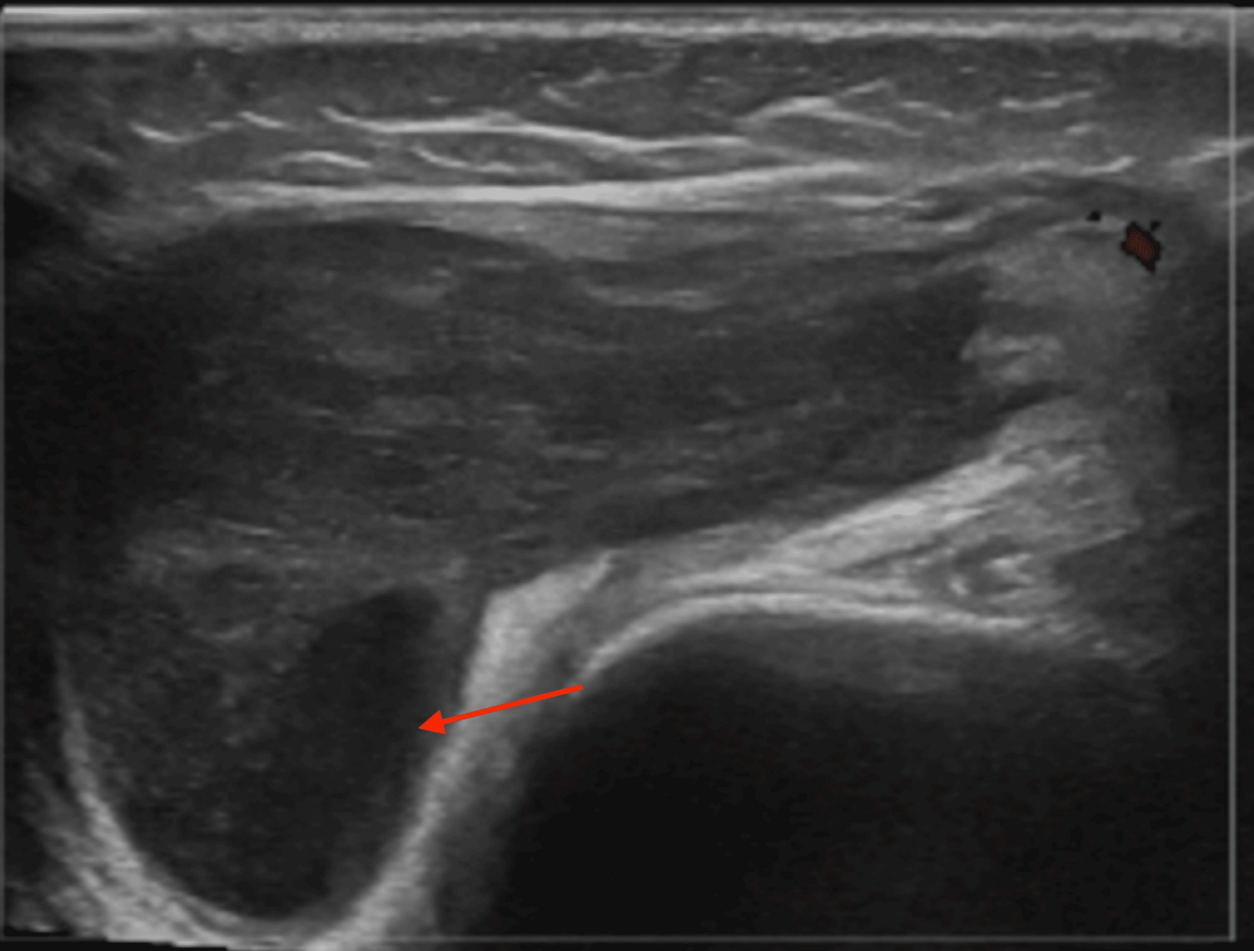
Ultrasound of the Right Knee Showing a Complex, Lobular Effusion Measuring 4.7x1.3x3.7 cm (Red Arrow)
